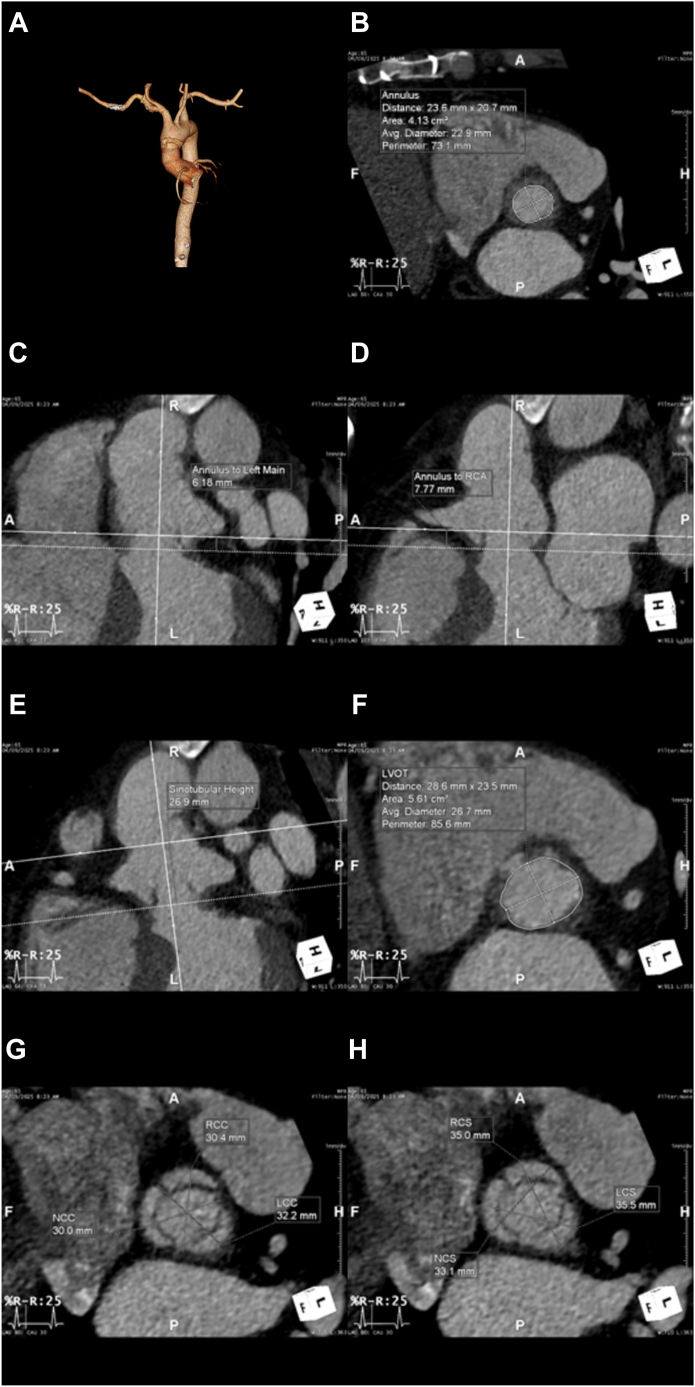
Preprocedural computed tomography (CT) angiography. (A) Multiplanar reconstruction from contrast-enhanced CT showing aortic root reconstructed with a Dacron graft from prior valve-sparing aortic root replacement. (B) The aortic annulus dimensions, with no significant annular calcification. (C-H) Coronary ostial heights, coronary sinus, and LVOT dimensions were adequate. LCC, left coronary cusp; LCS, left coronary sinus; LVOT, left ventricular outflow tract; NCC, non coronary cusp; NCS, noncoronary sinus; RCC, right coronary cusp; RCS, right coronary sinus.
